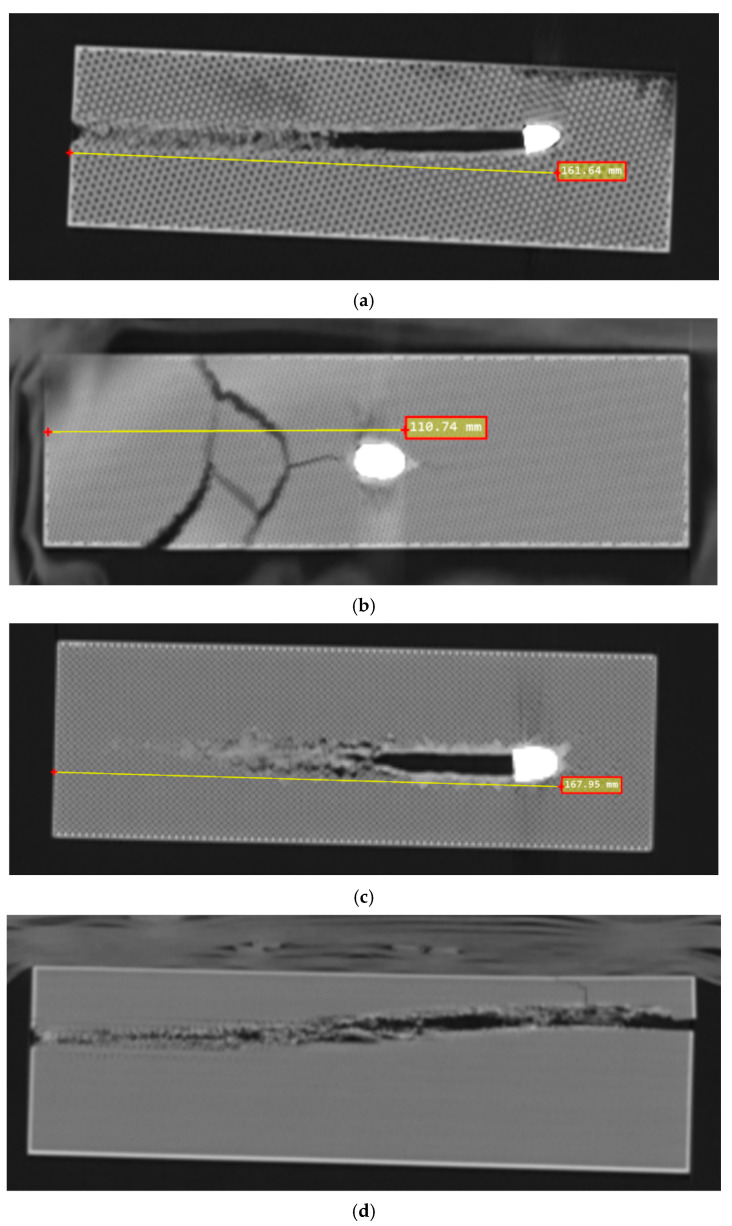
Representative CT scans for the four considered infill patterns: (a) cubic, (b) honeycomb, (c) grid, and (d) gyroid.
